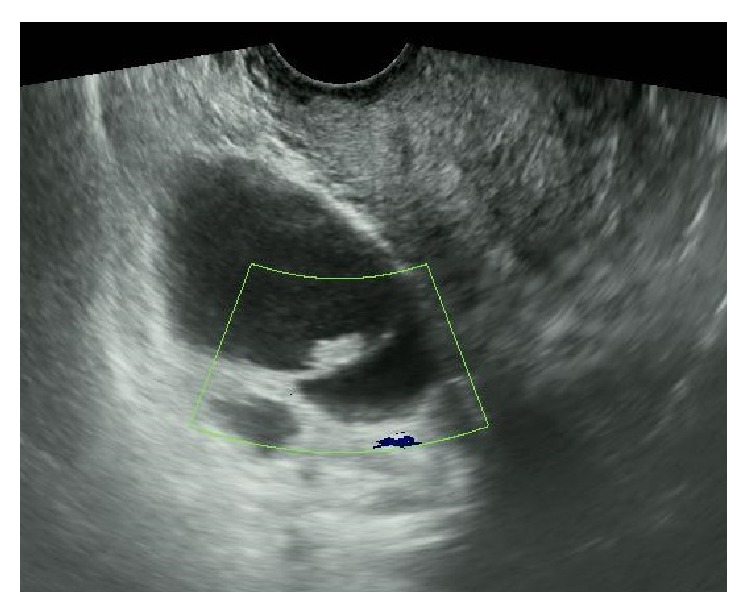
Ultrasonography demonstrated two cystic lesions in the right ovary. Color Doppler ultrasonography did not show neovascularity in the right ovary.
